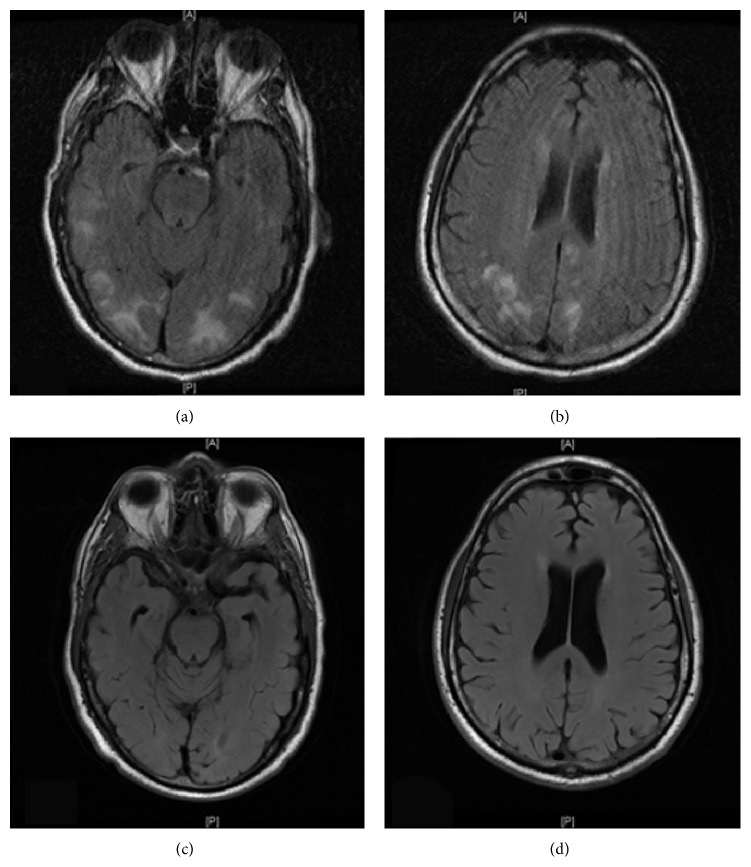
((a) and (b)) Brain MRI T2 FLAIR images show leptomeningeal enhancement and high signal intensity lesions in the subcortical white matter involving both hemispheres consistent with PRES. ((c) and (d)) Brain MRI T2 FLAIR images after 3 weeks show complete resolution of the lesions.
